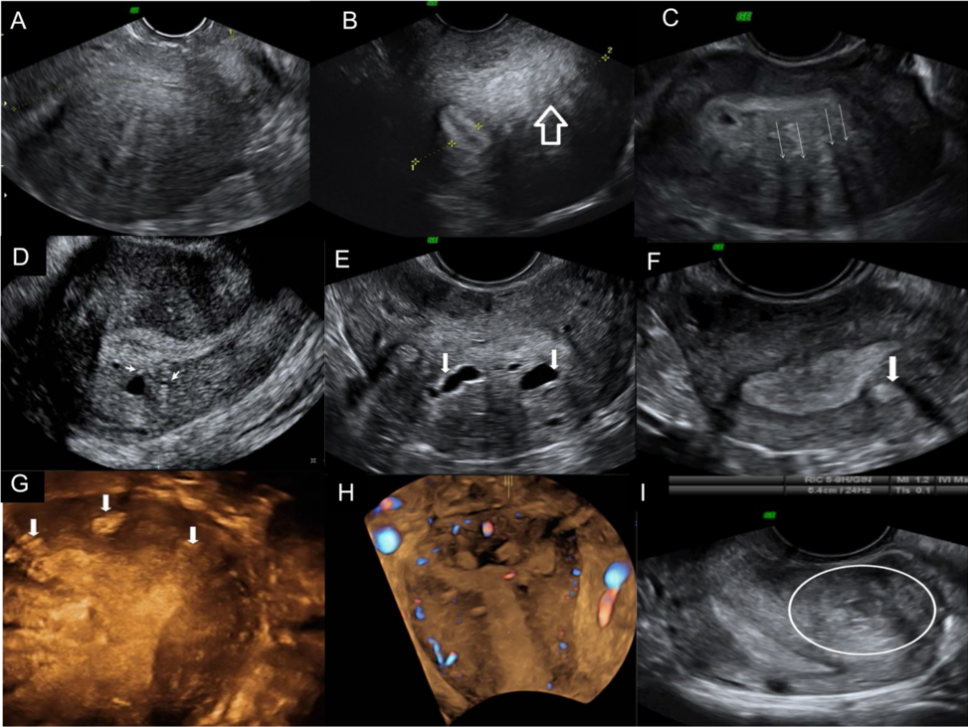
Ultrasonographic diagnostic criteria for adnomyosis. a Globulous aspect of the uterus. b Uterine asymmetry. Longitudinal section of a retroverted uterus, where the posterior uterine wall is clearly thicker than the anterior wall. c Heterogeneous myometrial texture. Transversal section of the uterus at the fundus level, where hypoechoic areas with radial pattern can be seen (*arrows*). d Linear striations. In this sagital section of an anteverted uterus thin hyperecogenic lines cross the myometrial thickness, visible from the endometrial-myometrial interphase. e Intramyometrial cysts. Transversal section of the uterus at the fundus level with sonoluscent images distributed in posterior wall of the myometrium. f and g, h Hyperechogenic nodules. Transversal (f) and coronal (g, h) sections of the uterus at the fundus level where hyperechogenic Intramyometrial areas can be observed (*arrows*). i Adenomyoma. Longitudinal section of a retroverted uterus with heterogeneous nodular mass lacking well-defined margins in the posterior wall
